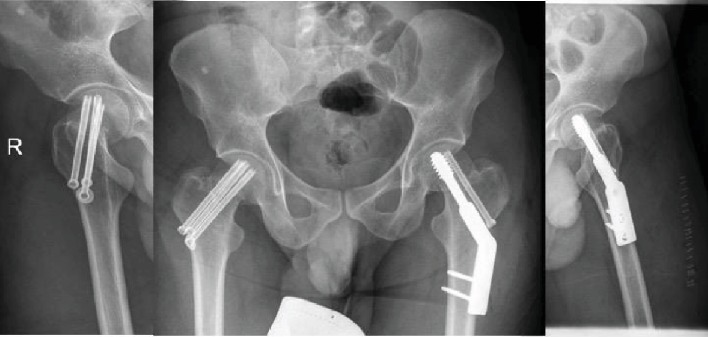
Immediate postoperative pelvis and hip radiographs.
